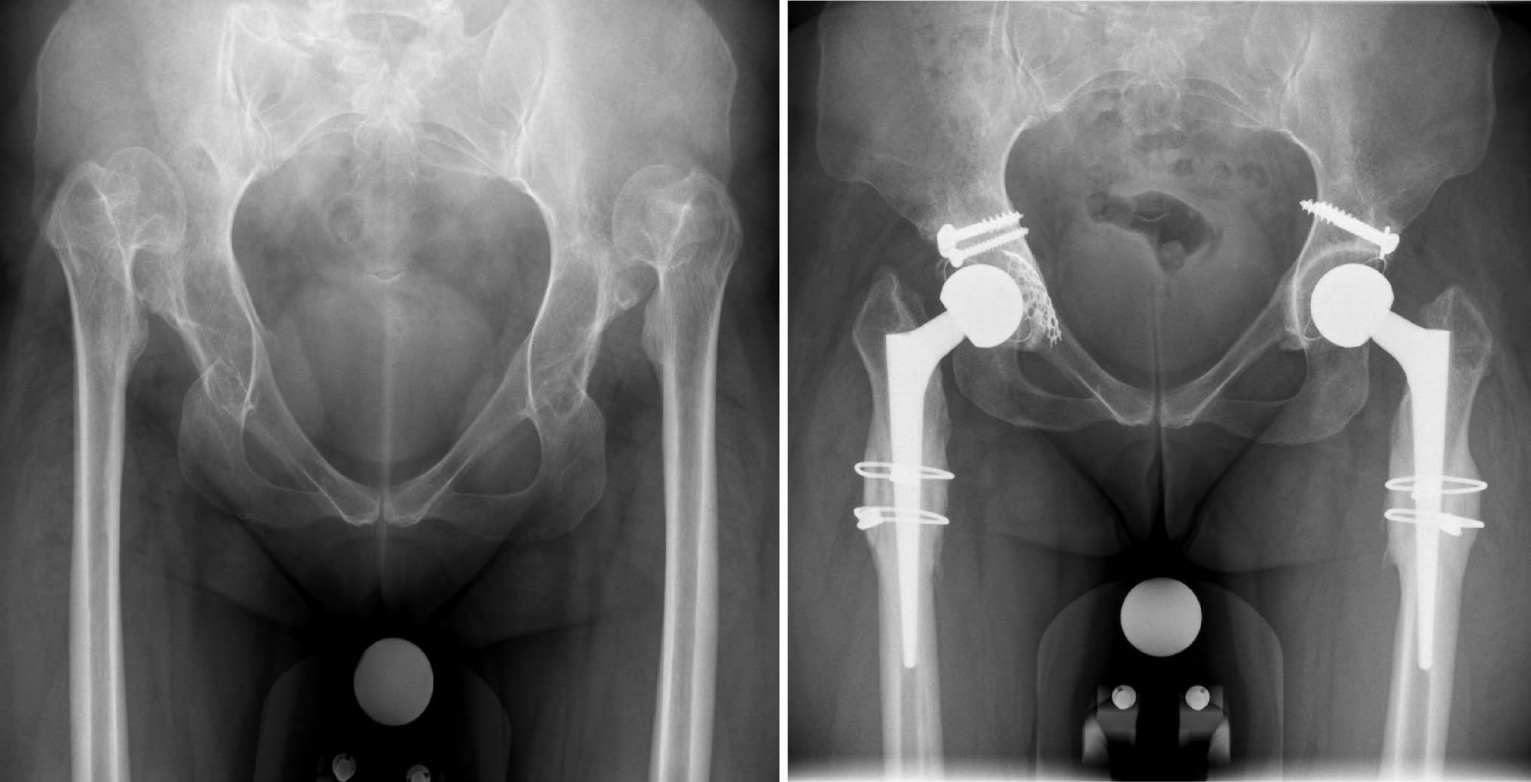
Pre- and postoperative X-ray of a patient with Crowe 4 DDH. The postoperative X-ray shows restoration of the original center of rotation (COR). In this patient bilateral massive autografts were placed and bilateral subtrochanteric osteotomy was performed
